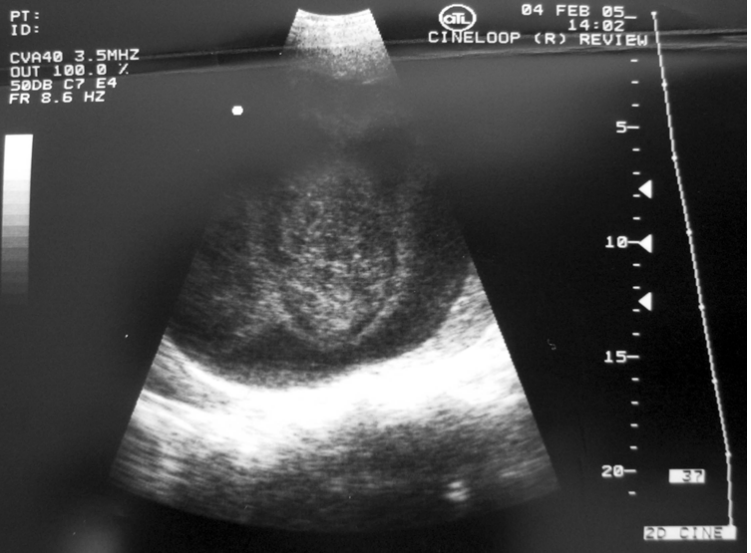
During second admission – 3 days post-admission. Ultrasound revealing the opaque fluid collection (abscess) inside the previously clear right liver lobe cystic mass.
